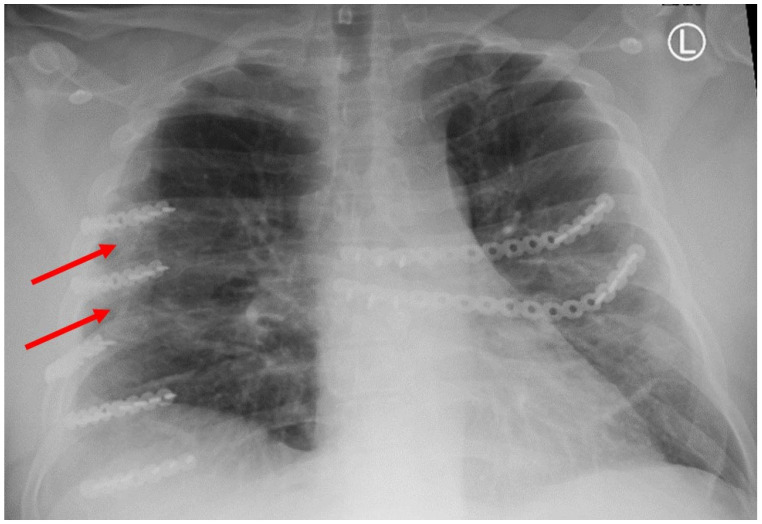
Chest X-ray showing HO at the tips of the red arrows.
